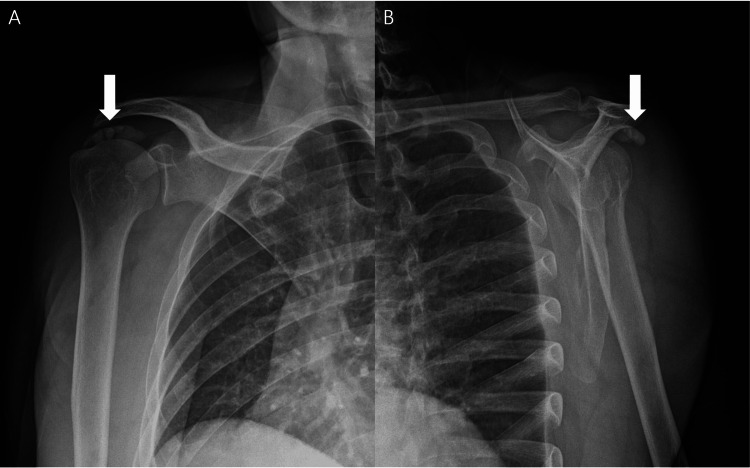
Large multilobulated calcific tendinitis of the right shoulder. (A) Anteroposterior radiograph of the right shoulder obtained at presentation shows a large, multilobulated calcific deposit measuring approximately 3 cm in the subacromial region (arrow), with a smaller adjacent calcification. (B) Scapular Y-view radiograph obtained at the same visit confirms the location of the calcific deposits in the subacromial region (arrow).
